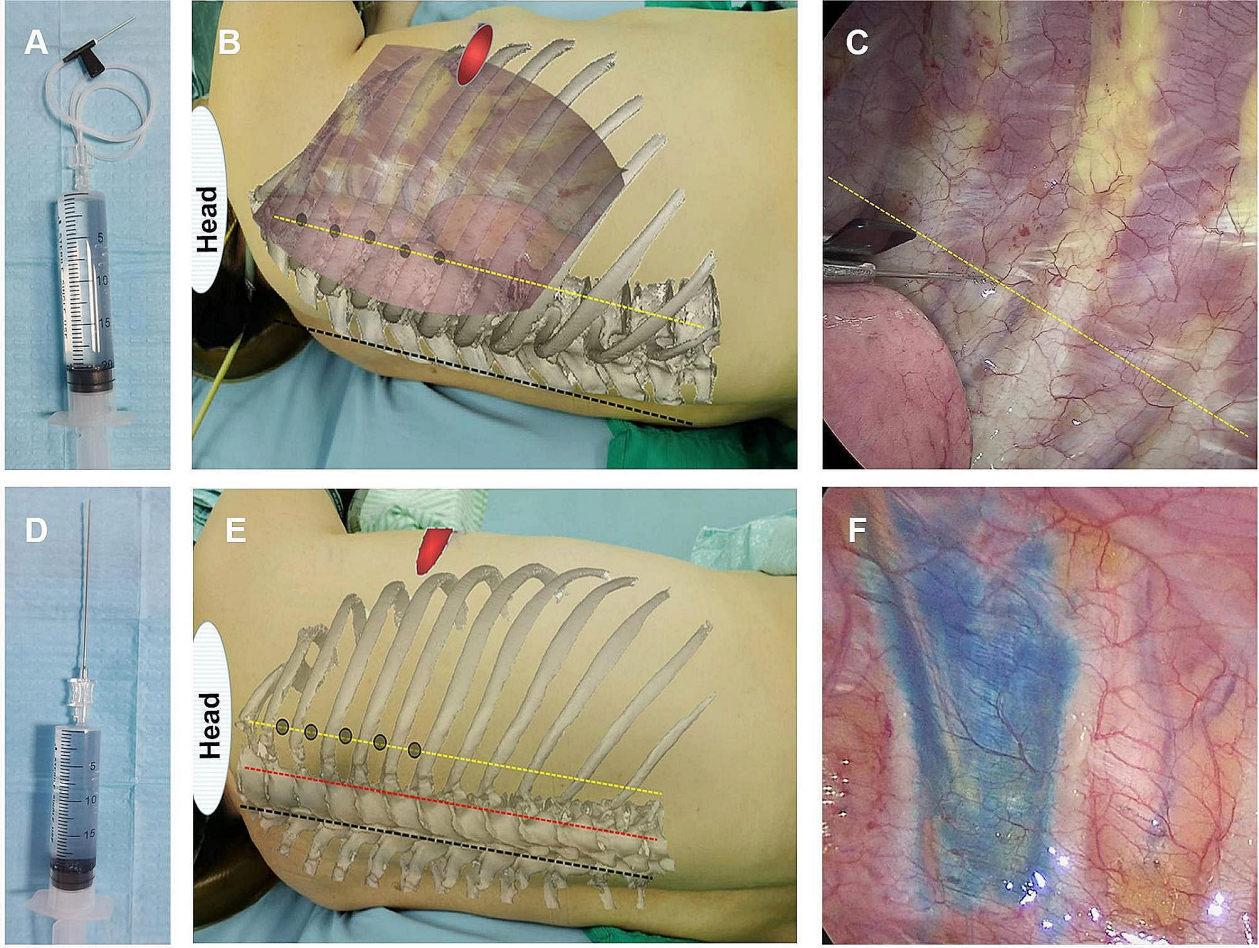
Transthoracic intercostal nerve block and percutaneous intercostal nerve block. a disposable intravenous infusion needle; b transthoracic intercostal nerve block sketch map, black line indicates posterior median line, yellow line indicates connecting line at 2 cm outside the sympathetic chain; c thoracoscopic view, yellow line indicates connecting line at 2 cm outside the sympathetic chain; d disposable anesthesia needle; e percutaneous intercostal nerve block sketch map, black line indicates posterior median line, red line indicates parallel line at 2.5 cm outside the posterior median line, yellow line indicates parallel line at 5 cm outside the posterior median line; f thoracoscopic view
